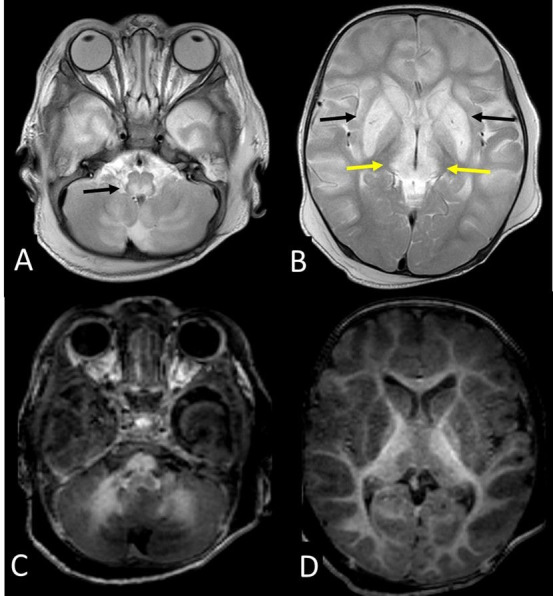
Axial T2-weighted (A and B) and T1-weighted (C and D) MR images show bilateral symmetric signal changes which were hyperintense on T2 and hypointense on T1-weighted images, involving brainstem dorsal tracts (black arrow, A), basal ganglia (black arrows, B), medial thalami (yellow arrows, B), with diffuse cerebral gyral swelling (more marked in fronto-temporal lobes) and cystic changes in bilateral putamina.
